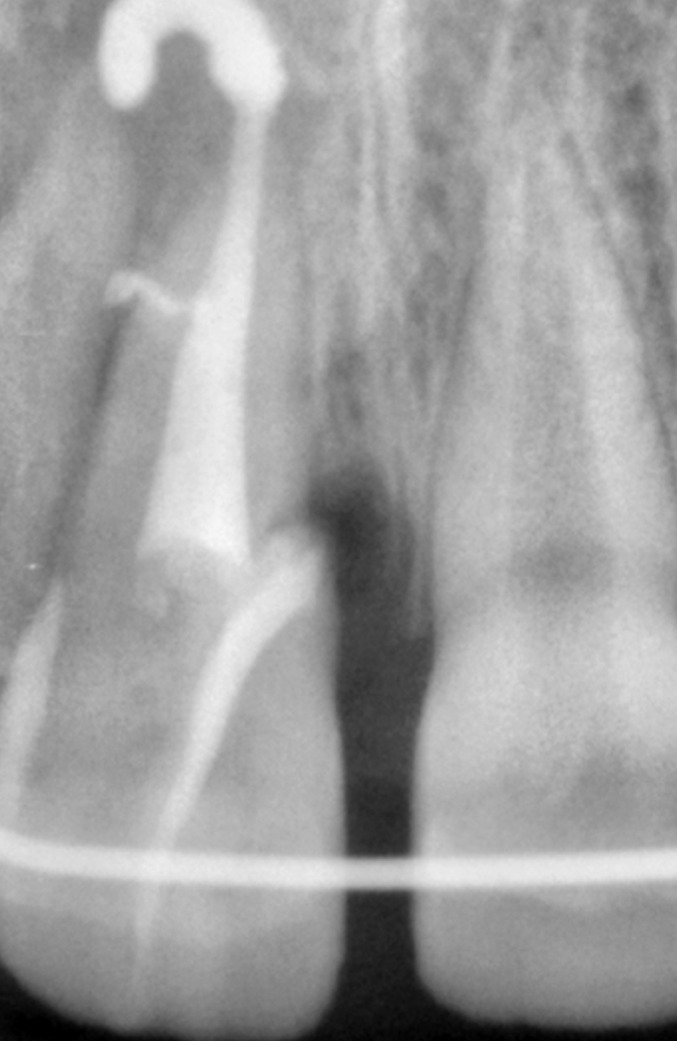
Postoperative radiograph of the involved tooth
